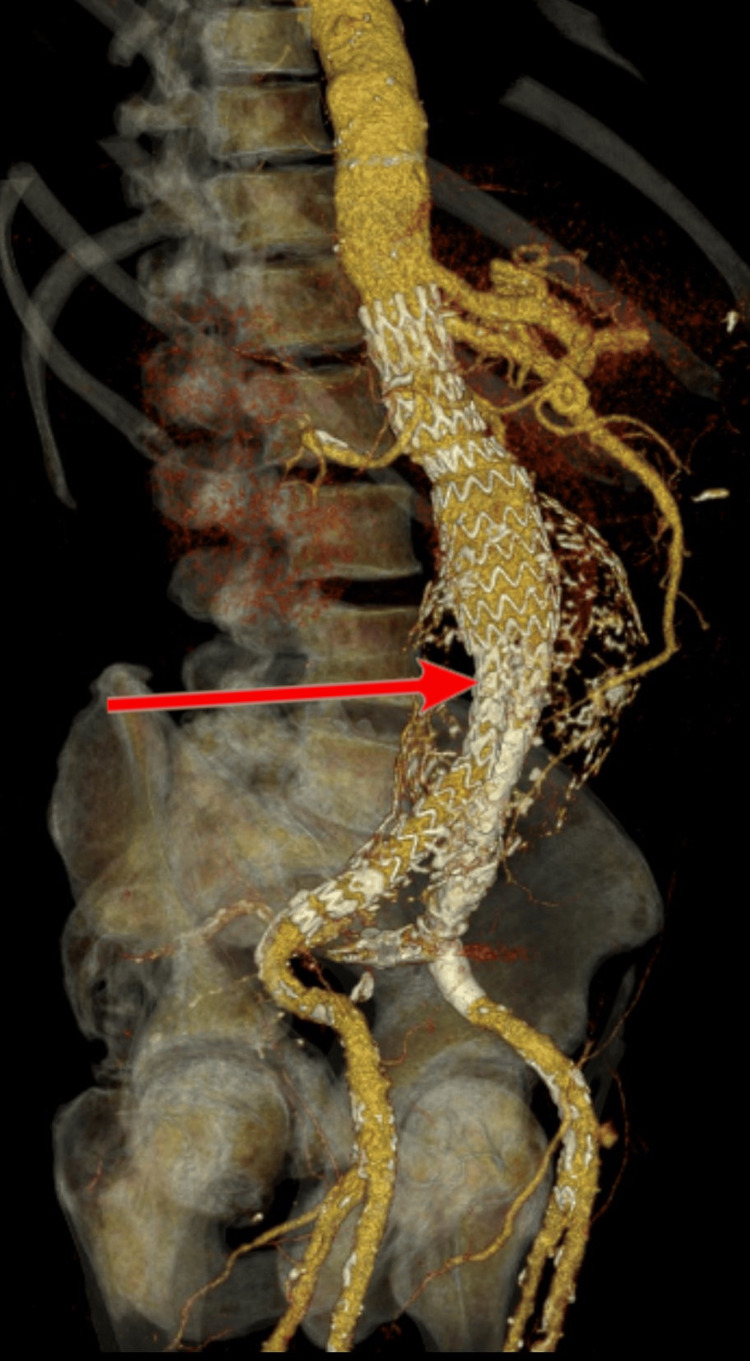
Computed tomography angiography control after endovascular aneurysm repair, stent graft position (arrow).
